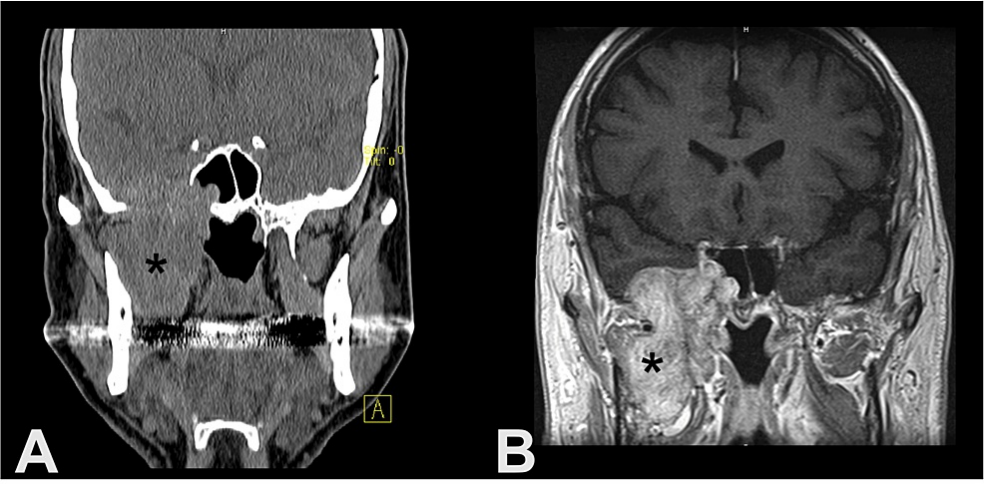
Image depicting RCC metastasis to the IT fossa, middle fossa, and sphenoid sinus (*) as seen on coronal CT of the head (A); image of RCC metastasis to the IT fossa, middle fossa, and sphenoid sinus (*) as seen on coronal MRI of the head (B). RCC: Renal cell carcinoma.
